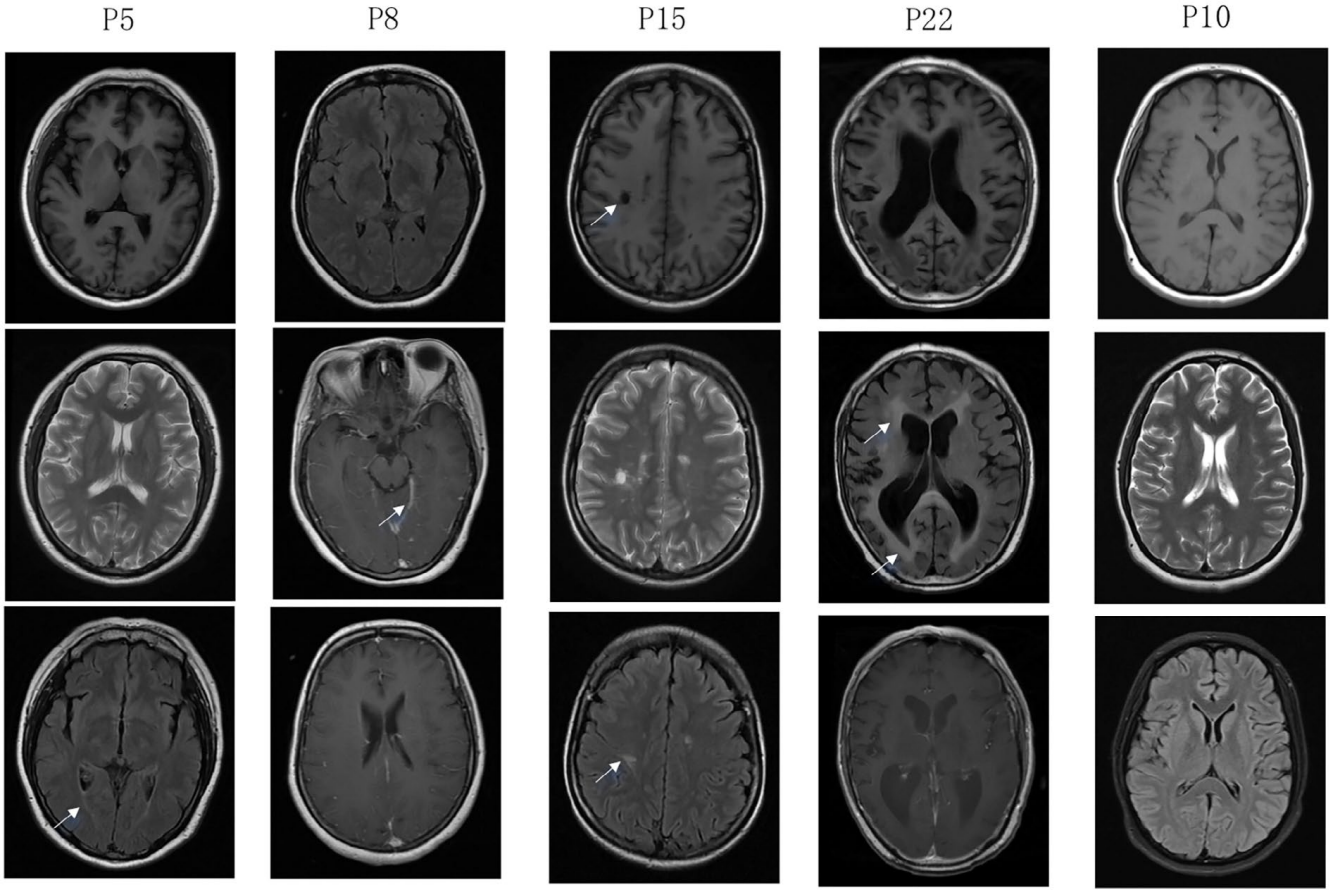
The image findings of the included patients. Cranial MRI of P5 revealed mild white matter degeneration (indicated by arrow). Cranial MRI of P8 suggested thickening of the bilateral cerebellar tentorium. In P15, multiple abnormal signals were observed in T1, T2, and FLAIR, suggesting demyelinating changes (indicated by arrows). Diffuse white matter changes were observed in the periventricular white matter (indicated by arrows) without enhancement in P22's cranial MRI. Cranial MRI of P10 was normal. FLAIR, fluid‐attenuated inversion recovery; MRI, magnetic resonance imaging.
